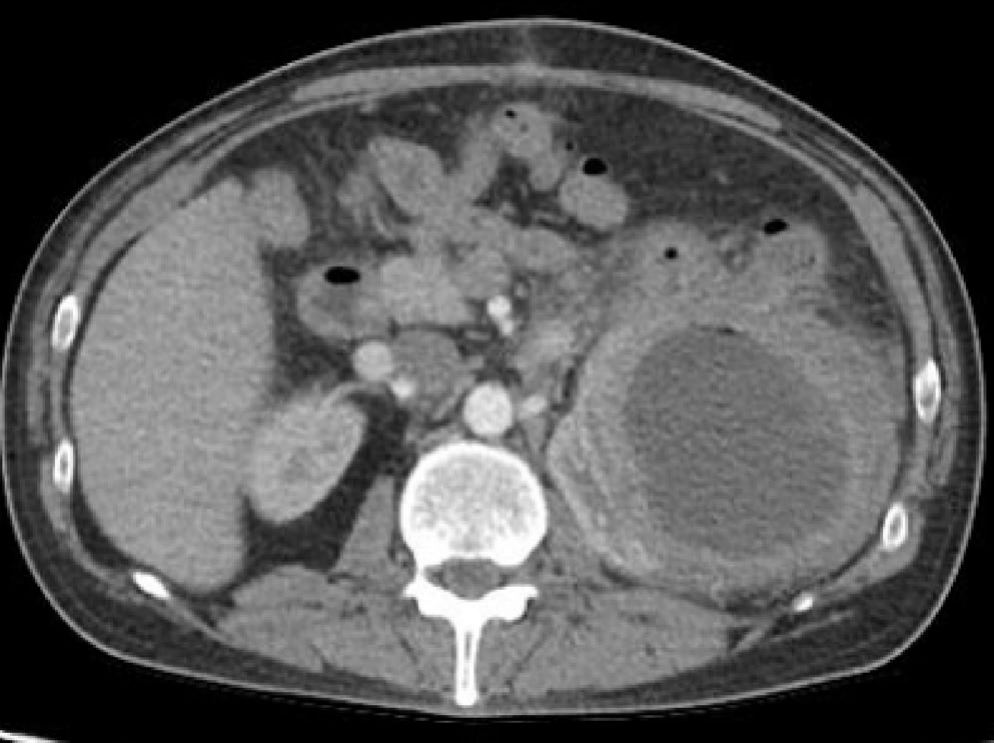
Follow‐up CT scan showed a decrease in the size of the peripancreatic pseudocyst with development enhancing thick walls along with surrounding fat stranding.
